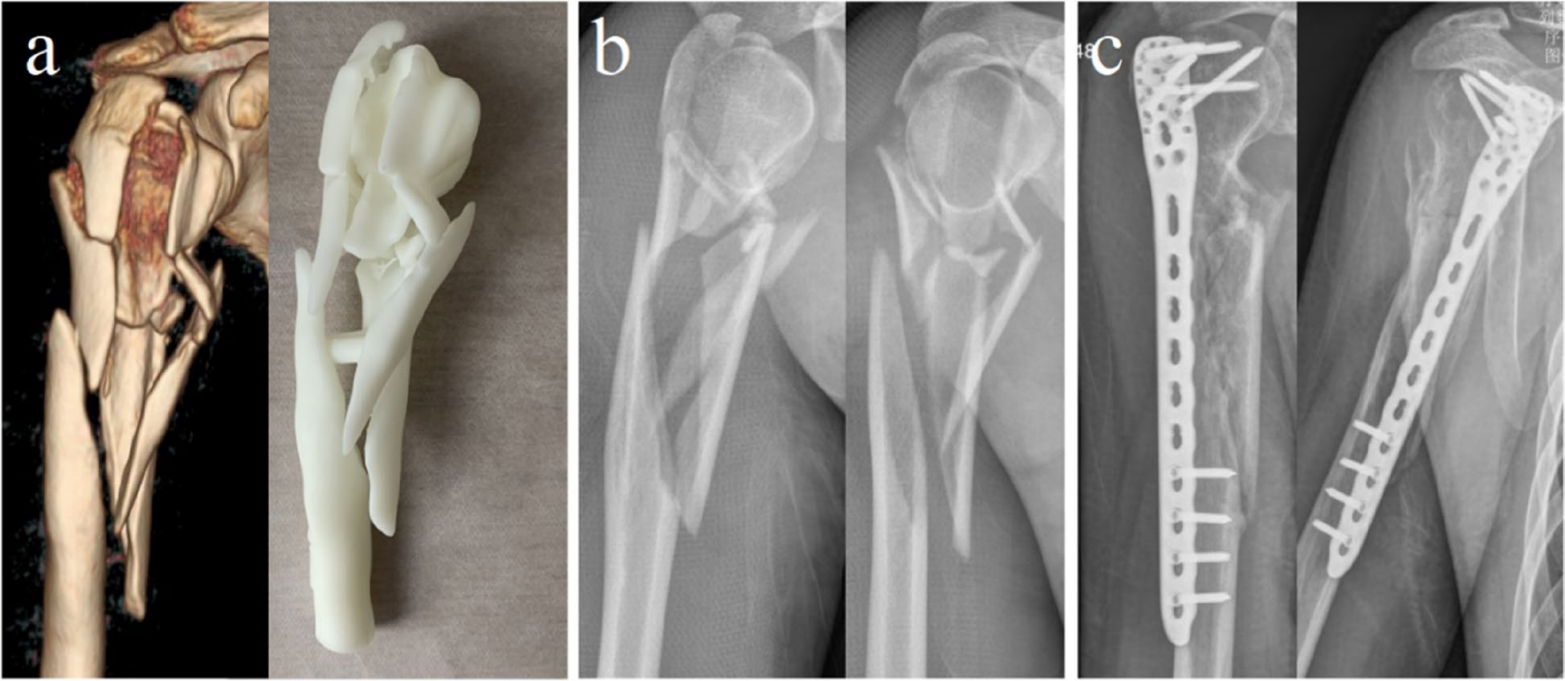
Case 3: a 33-year-old male, the AO/OTA classification was 12C13. a Preoperative 3D CT image and 3D anatomical model of middle-proximal humeral fracture. b Preoperative X-ray radiograph of the middle-proximal humerus fractures. c The X-ray radiograph of full-length humerus 4 months after MIPO
